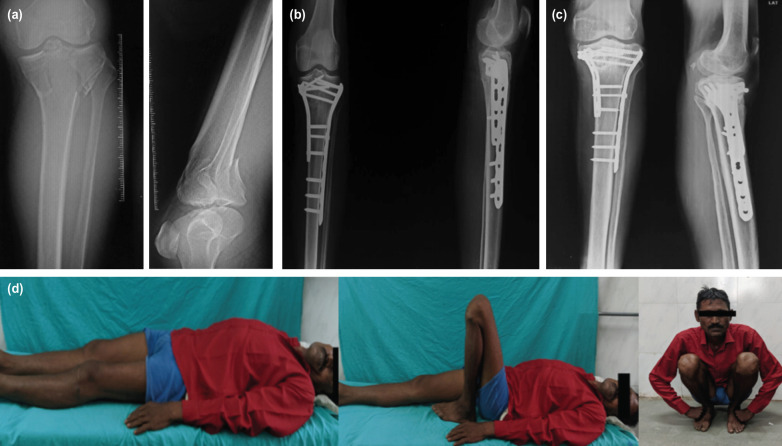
(a) Radiograph showing bicondylar tibial plateau fracture. (b) Post-operative radiograph showing fixation with dual plating. (c) Six months post-operative radiograph showing union. (d) Clinical pictures showing good range of movement.
